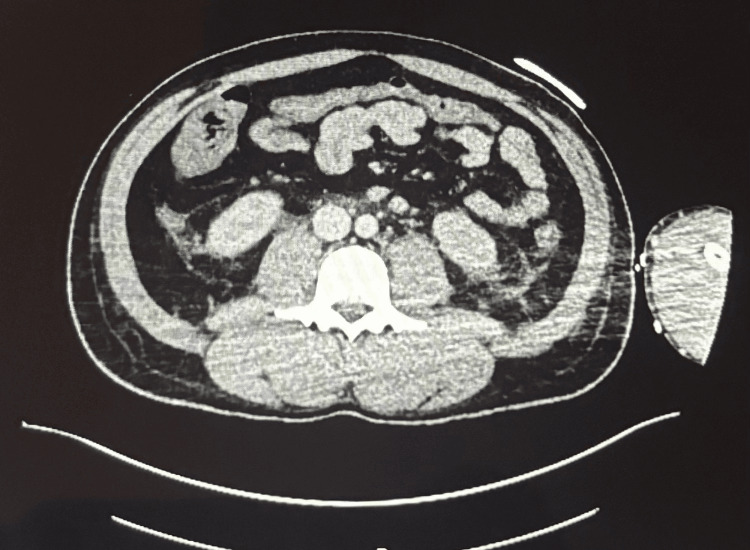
CT abdomen showing mild acute colitis from the ascending to descending colon CT: computed tomography
